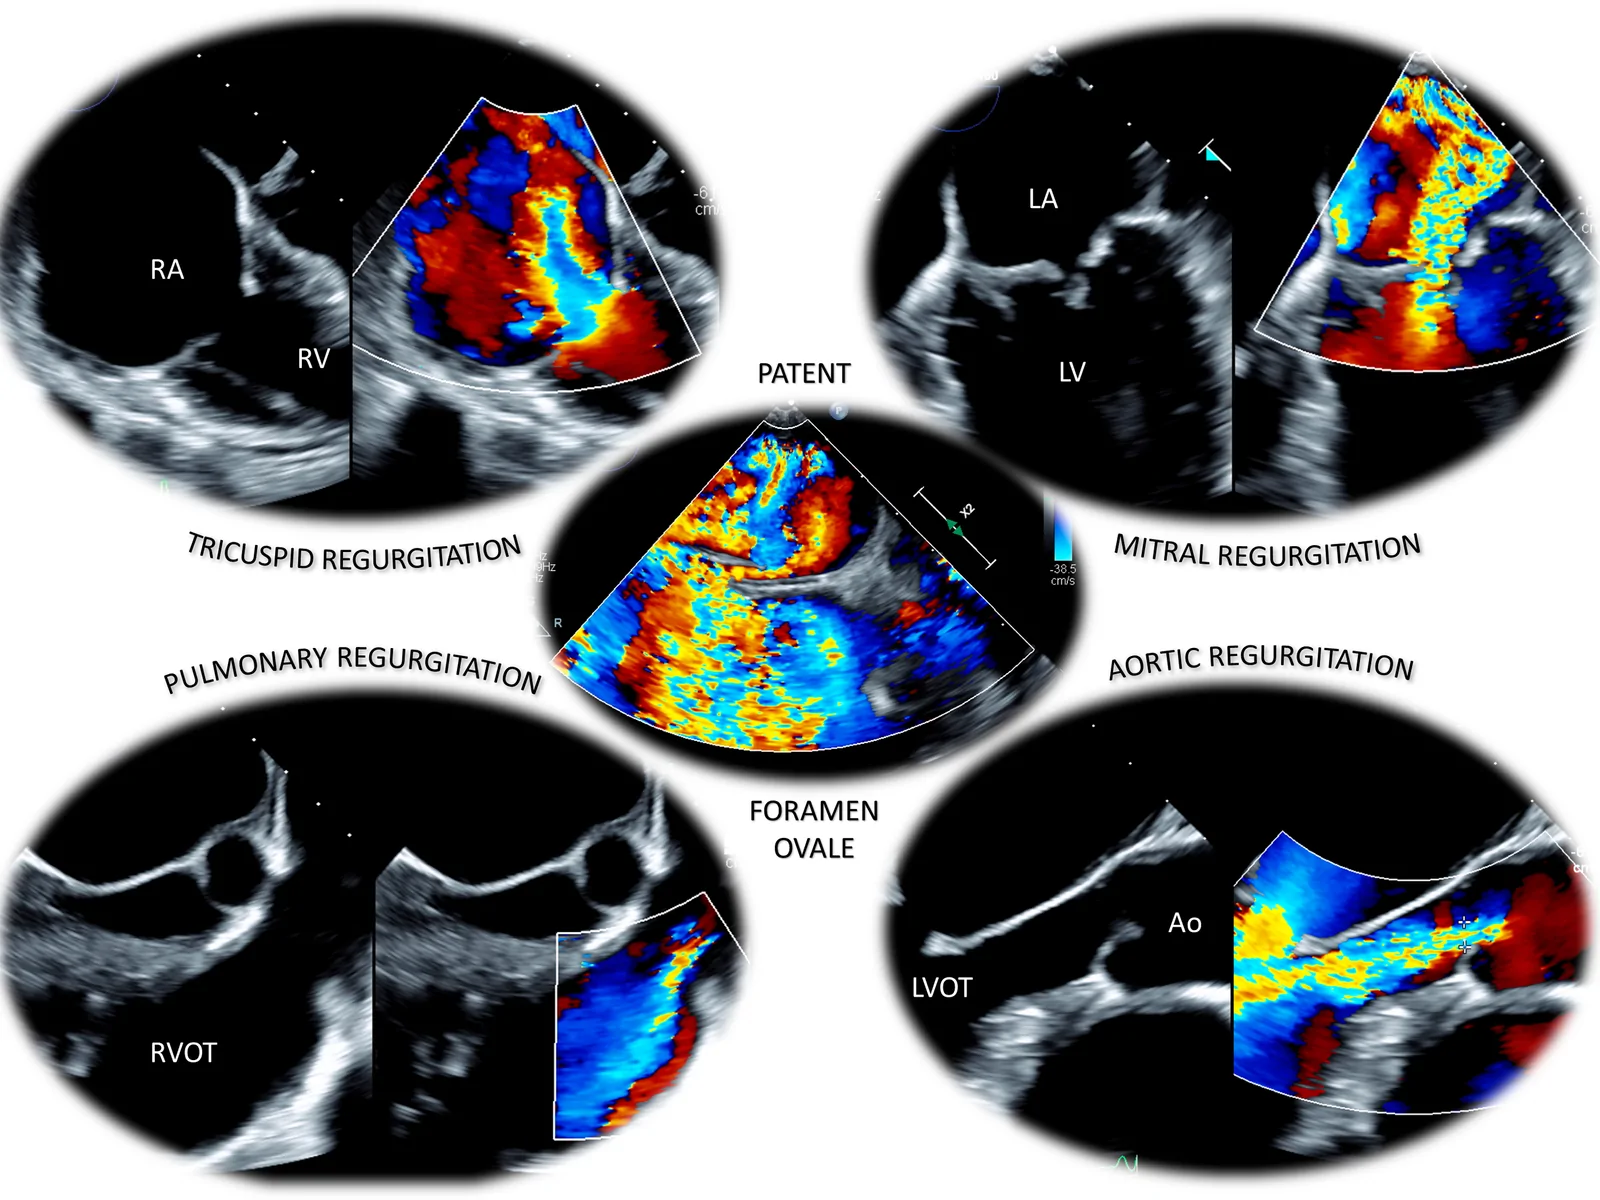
Transesophageal Echocardiography Images Showing Severe Regurgitation of All 4 Valves and a Bidirectional Patent Foramen Ovale Ao = aorta; LVOT = left ventricular outflow tract; other abbreviations as in Figure 2.
